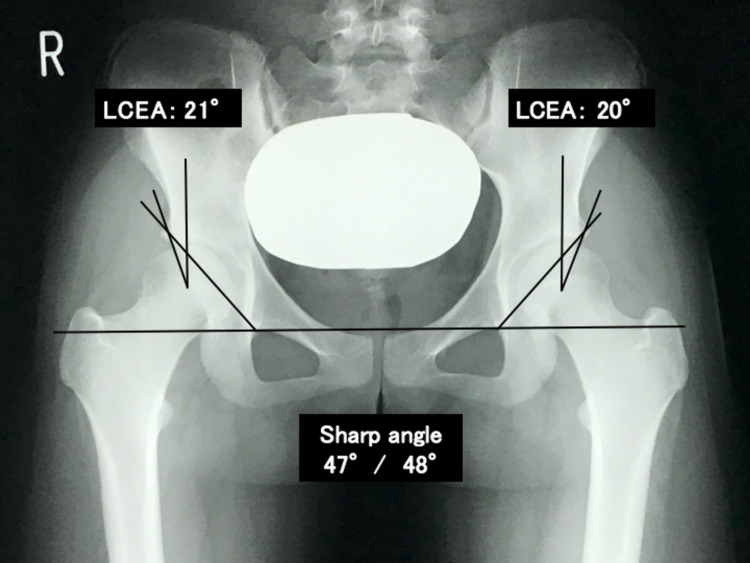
Radiograph, anteroposterior view, shows no findings of osteoarthritis. LCEA: lateral center-edge angles
